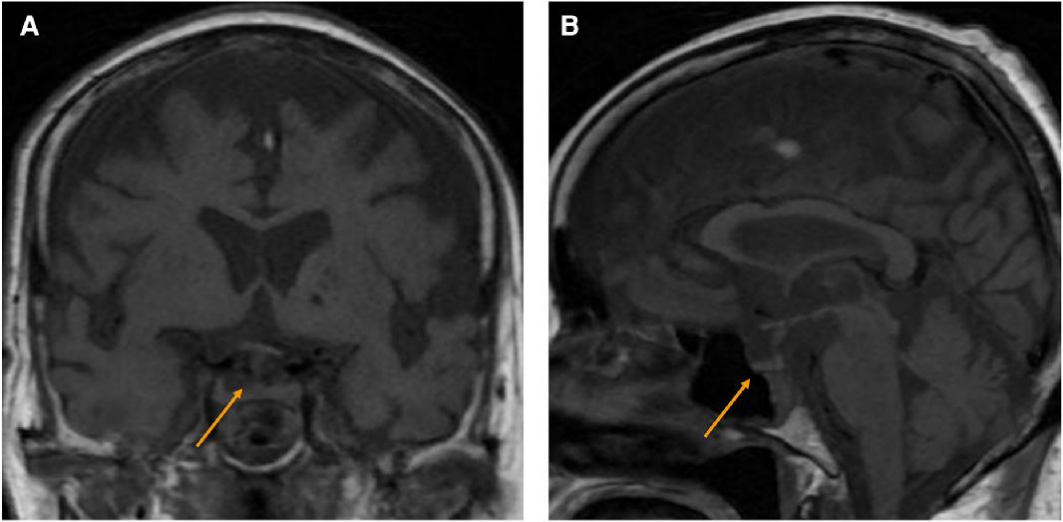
Magnetic resonance imaging findings of the sella turcica obtained 8 weeks after hospitalization. A, Sagittal and B, coronal T1-weighted images obtained 8 weeks after hospitalization showed no abnormalities in the pituitary gland.
